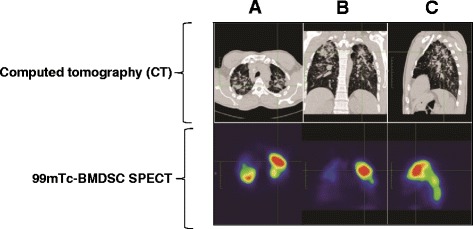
Representative image of CT and 99mTc-BMDSC SPECT showing correspondence of a left lung lesion (green triangular area). CT, computed tomography; 99mTc-BMDSC, 99mTc-labeled bone marrow–derived mononuclear cells; SPECT, single-photon emission computed tomography
